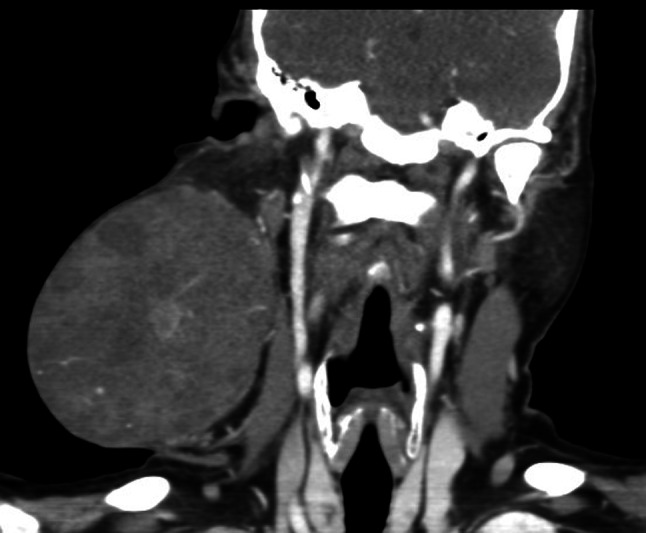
Coronal CT neck - showing tumour pre-operation
